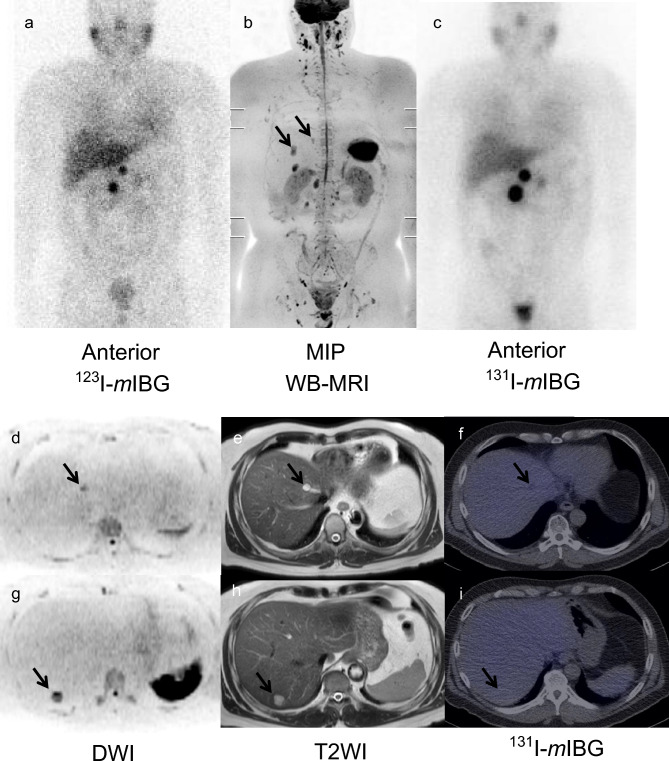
A case of WB-MRI confusing liver metastases with benign hepatic lesions (patient No. 9). A male patient in his 50s with multiple bone metastases of pheochromocytoma. Liver metastases, in addition to the metastases, were suspected by WB-MRI alone (arrows). Abnormal signal density and structures of DWI and T2WI revealed liver cysts, with no abnormal accumulation on post-therapy ^131^I-*m*IBG images. (a) ^123^I-*m*IBG scintigram (anterior). (b) The MIP of WB-MRI. (c) Post-therapy ^131^I-*m*IBG scintigram (anterior). (d,g) DWI (axial). (e,h) ^123^I-*m*IBG SPECT/CT (axial). (f,i) Post-therapy ^131^I-*m*IBG SPECT/CT (axial). *WB-MRI* whole-body magnetic resonance image, *MIP* maximum intensity projection, *DWI* diffusion-weighted image, *T2WI* T2 weighted image, *mIBG meta*-iodo-benzylguanidine.
